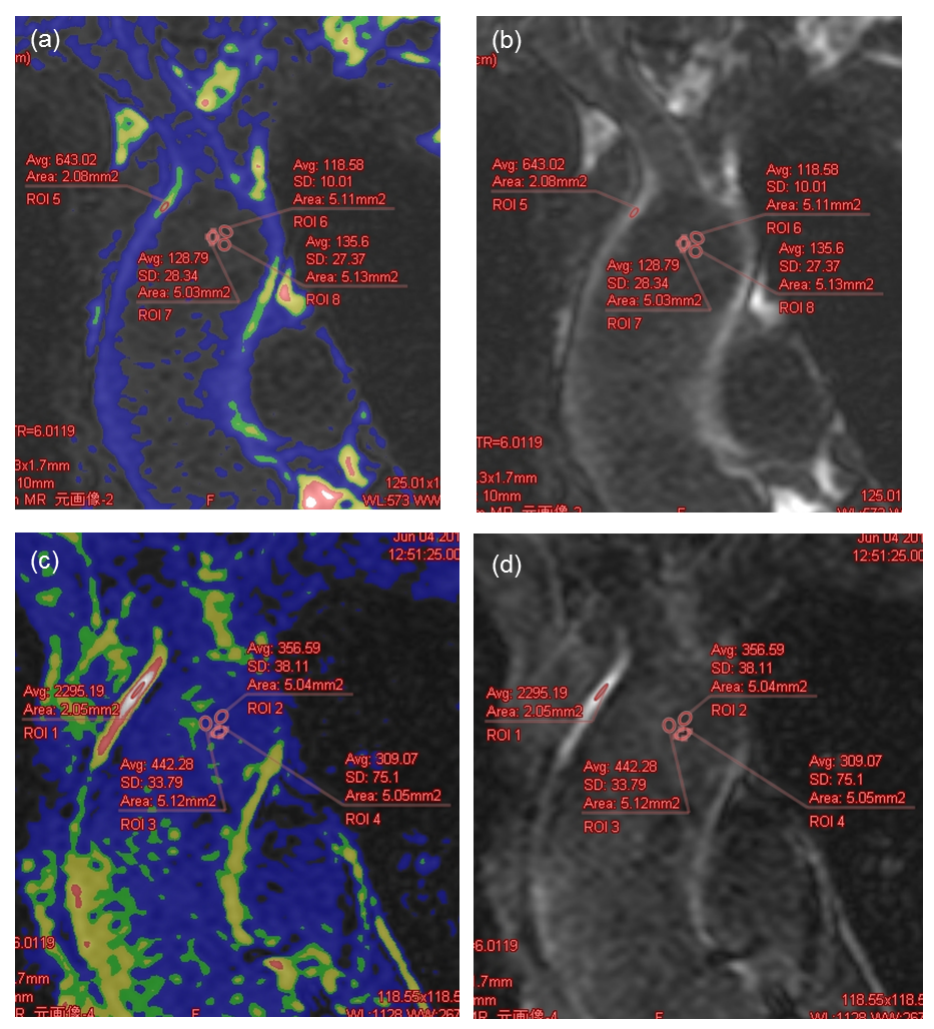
MRI imaging. (a) Representative pre-contrast image with color map. (b) Representative pre-contrast image. (c) Representative post-contrast image with color map. The site of the aortic wall site with the highest SI was determined using this image. (d) Representative post-contrast image. SI, signal intensity; LGE, late gadolinium enhancement.
